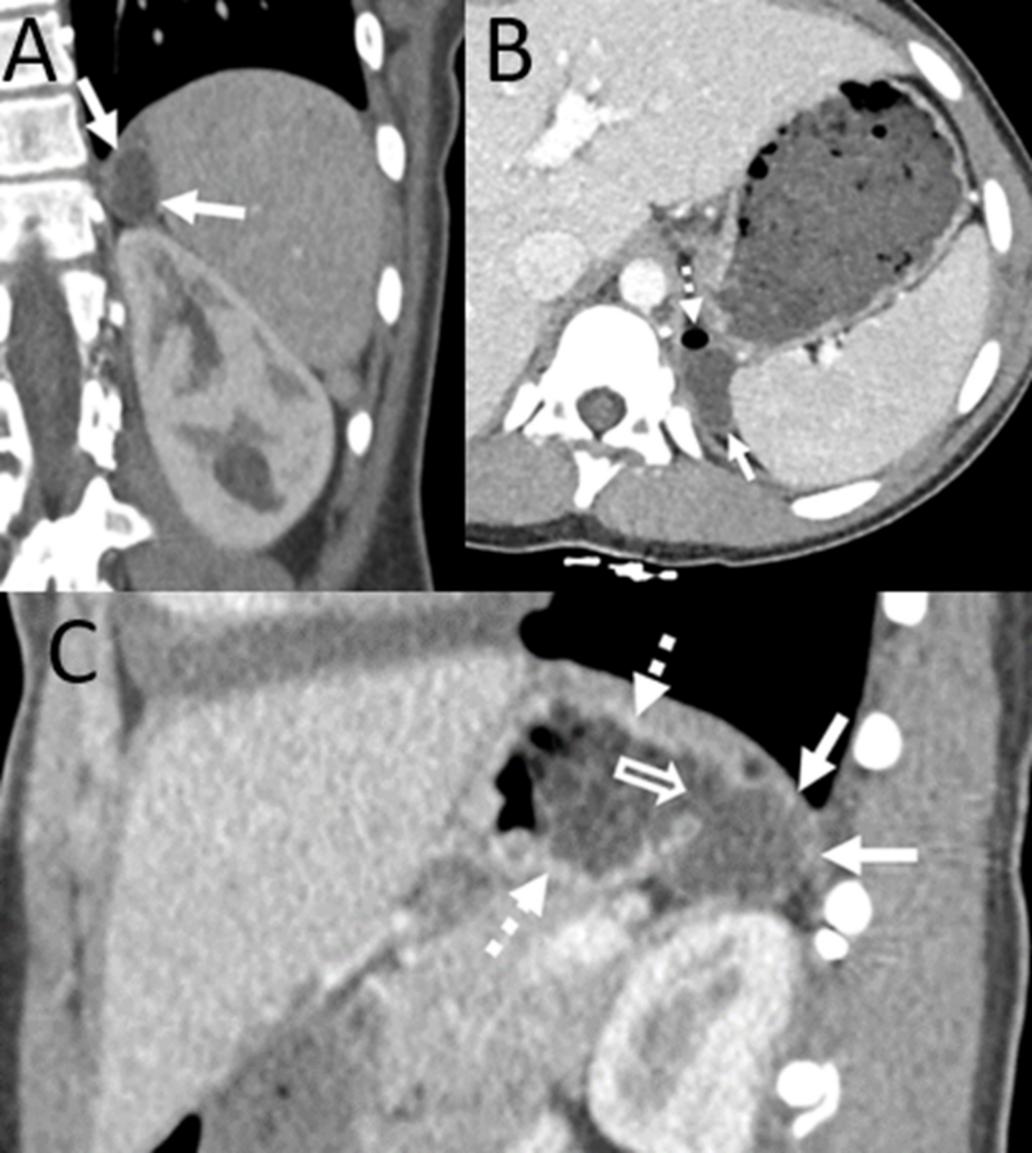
CT scan of the abdomen with IV contrast performed seven months earlier. Coronal (A), axial (B) and sagittal (C) images show the gastric diverticulum (solid arrows). Note the air along the non-dependent aspect of the diverticulum on the axial (B) image (dashed arrow). The sagittal image (C) clearly shows a connection (open arrow) between the diverticulum (solid arrows) and the fundus of the stomach (dashed arrows).
